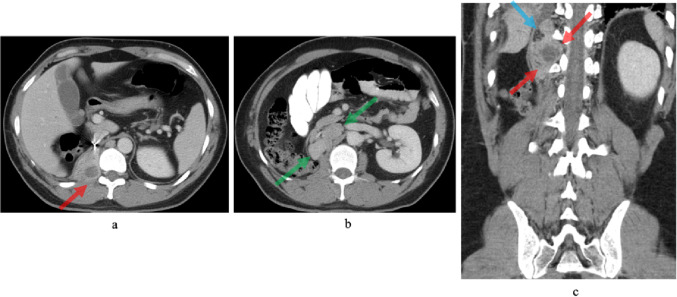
Retroperitoneal AML. Axial (a) and coronal (c) contrast-enhanced CT images show a heterogeneously enhancing right retrocrural mass (red arrows) with a small fat containing component (blue arrow). An axial (b) contrast-enhanced CT image through the mid-abdomen shows enhancing soft tissue masses in the retrocaval and aortocaval regions (green arrows). Surgical clips are from prior right nephrectomy
